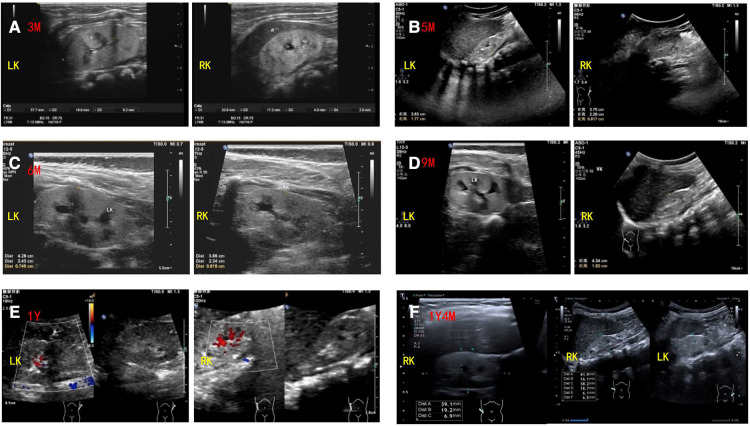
Renal ultrasound findings of the patient. Ultrasound showed bilateral hypodysplastic kidneys with enhanced echogenicity and obtained corticomedullary differentiation. The size and thickness of left and right kidneys of the patient are as follows: (A) 3 months: 38 mm × 19 mm, 6.2 mm and 34 mm × 17 mm, 4.8 mm (reference range: 55.7–64.1 mm × 32.2–36.2 mm, 23.1–28.1 mm and 55.4–63.8 mm × 29.4–33.4 mm, 22.3–26.9 mm); (B) 5 months: 38 mm × 22 mm, 8.1 mm and 36 mm × 17 mm, 6.4 mm (reference range: 55.7–64.1 mm × 32.2–36.2 mm, 23.1–28.1 mm and 55.4–63.8 mm × 29.4–33.4 mm, 22.3–26.9 mm); (C) 6 months: 43 mm × 24 mm, 7.4 mm and 41 mm × 21 mm, 8.0 mm (reference range: 55.7–64.1 mm × 32.2–36.2 mm, 23.1–28.1 mm and 55.4–63.8 mm × 29.4–33.4 mm, 22.3–26.9 mm); (D) 9 months: 45 mm × 25 mm, 7.4 mm and 43 mm × 21 mm, 7.6 mm (reference range: 62.3–67.3 mm × 34.2–37.2 mm, 24.9–29.7 mm and 61.6–67.4 mm × 33.7–37.3 mm, 23.7–30.1 mm); (E) 1 year: 41 mm × 19 mm, 8.1 mm and 42 mm × 18 mm, 6.8 mm (reference range: 62.3–67.3 mm × 34.2–37.2 mm, 24.9–29.7 mm and 61.6–67.4 mm × 33.7–37.3 mm, 23.7–30.1 mm); (F) 1 year and 4 months: 40 mm × 19 mm, 6.1 mm and 41 mm × 19 mm, 6.5 mm (reference range: 62.3–67.3 mm × 34.2–37.2 mm, 24.9–29.7 mm and 61.6–67.4 mm × 33.7–37.3 mm, 23.7–30.1 mm).
